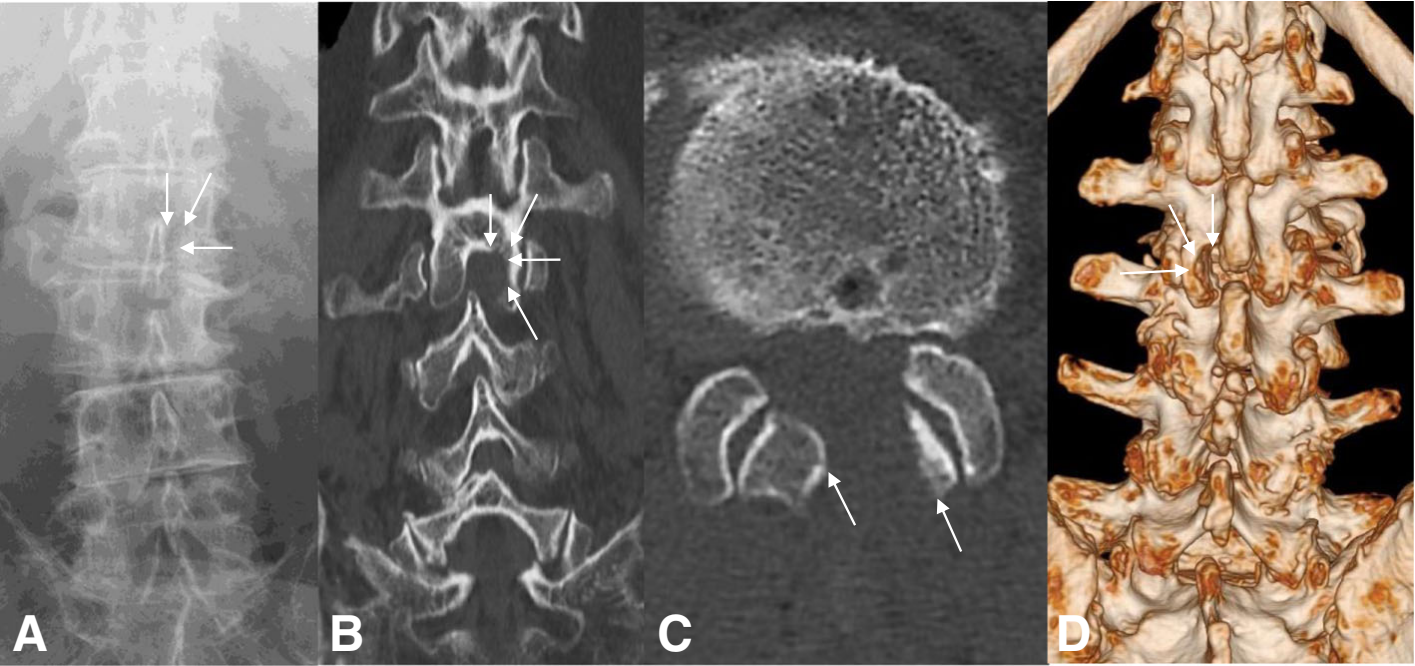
X-ray, computed tomography, and reconstruction three-dimensional computed tomography shows the interlaminar window open and the facet joint preserved. a X-ray. b Coronal view. c Axial view. d Three-dimensional computed tomography. The *arrows* are pointing to the interlaminar window
